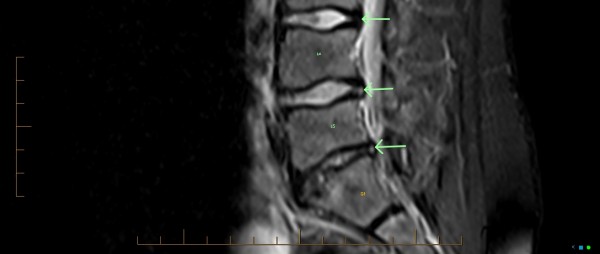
MRI, sagittal STIR midline image. Annular tears are seen as posterior annulus fibrosus hyperintensity (bottom two arrows pointing to white dots) at L4/5 and L5/S1 discs. Compare with low signal (dark) of the normal L3/4 disc (top arrow).
